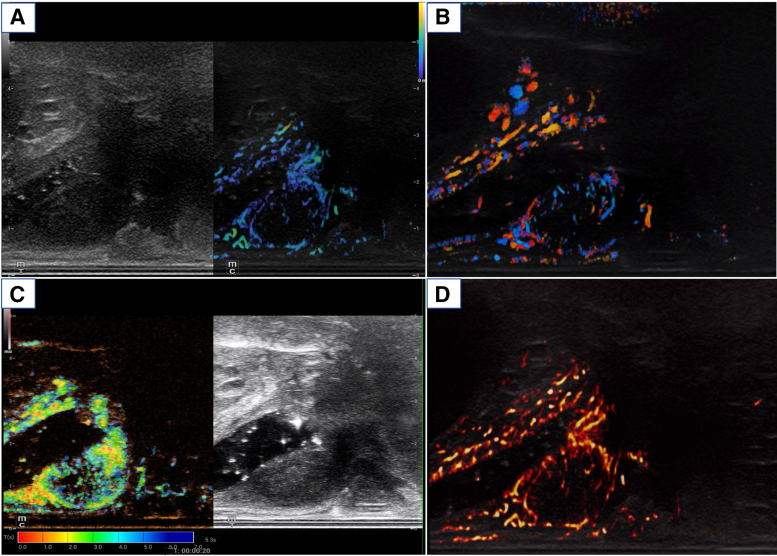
SR CEUS images. (A) Blood flow velocity map; (B) Blood flow direction map; (C) Temporal pattern map; (D) Vascular density map. SR CEUS = super resolution contrast-enhanced ultrasound.
